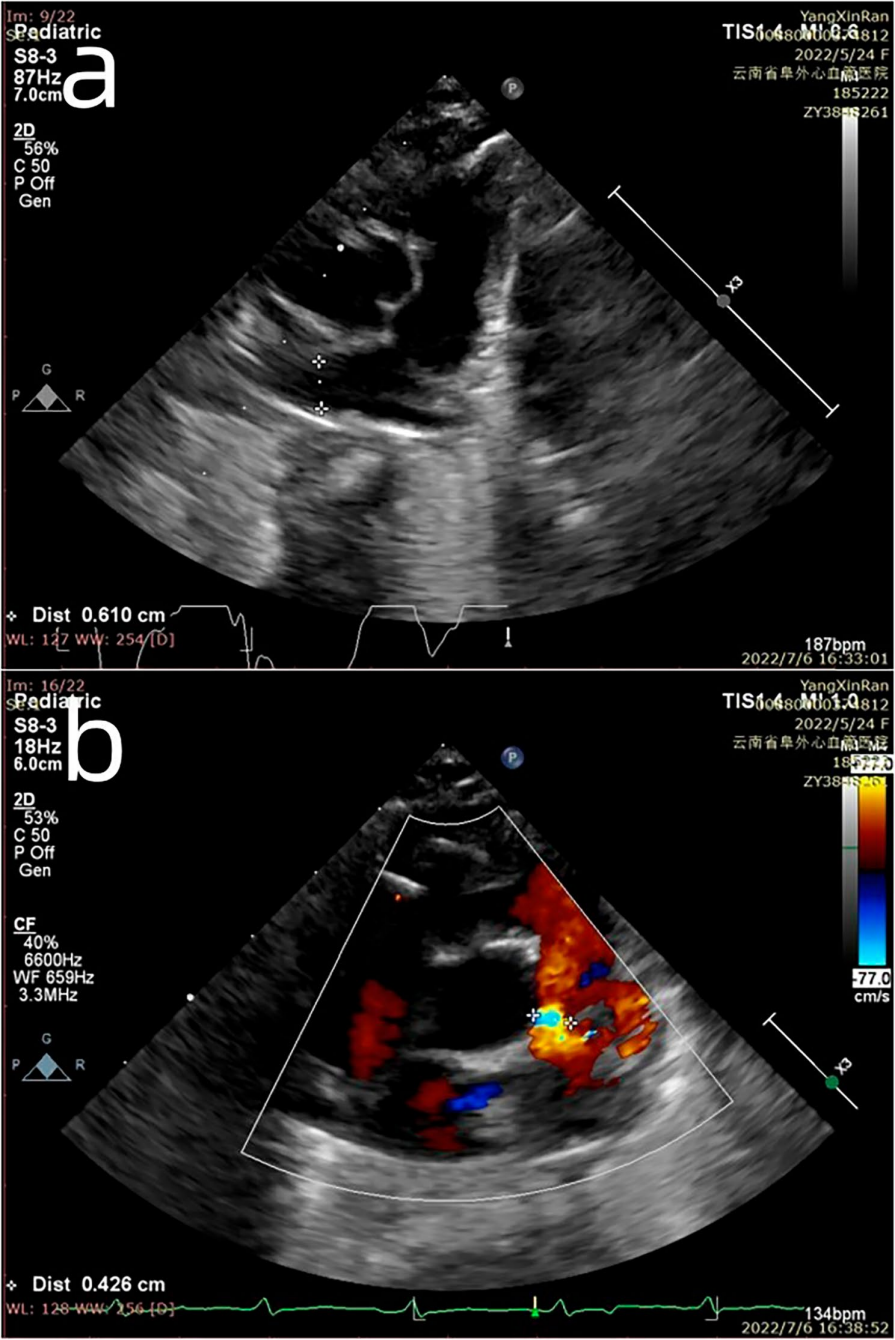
Post-operative ultrasound showed satisfactory pulmonary arterioplasty (a) with mild to moderate pulmonary valve regurgitation (b)
